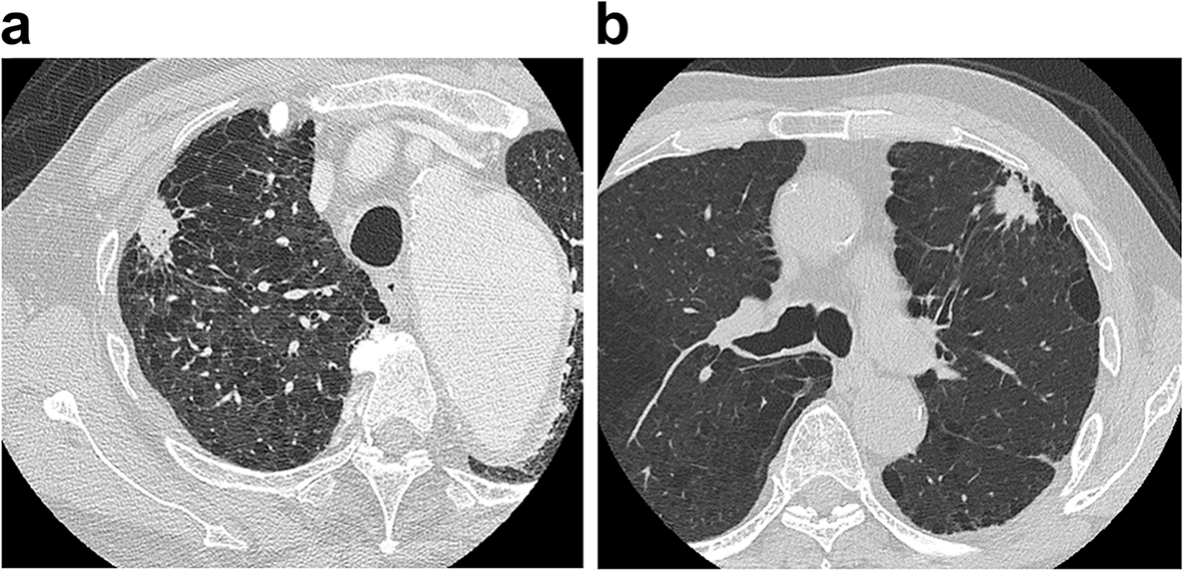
Lung cancer in midst of emphysema and/or interface of emphysema and normal lung. These are examples of computed tomography findings for lung cancers in midst of emphysema
